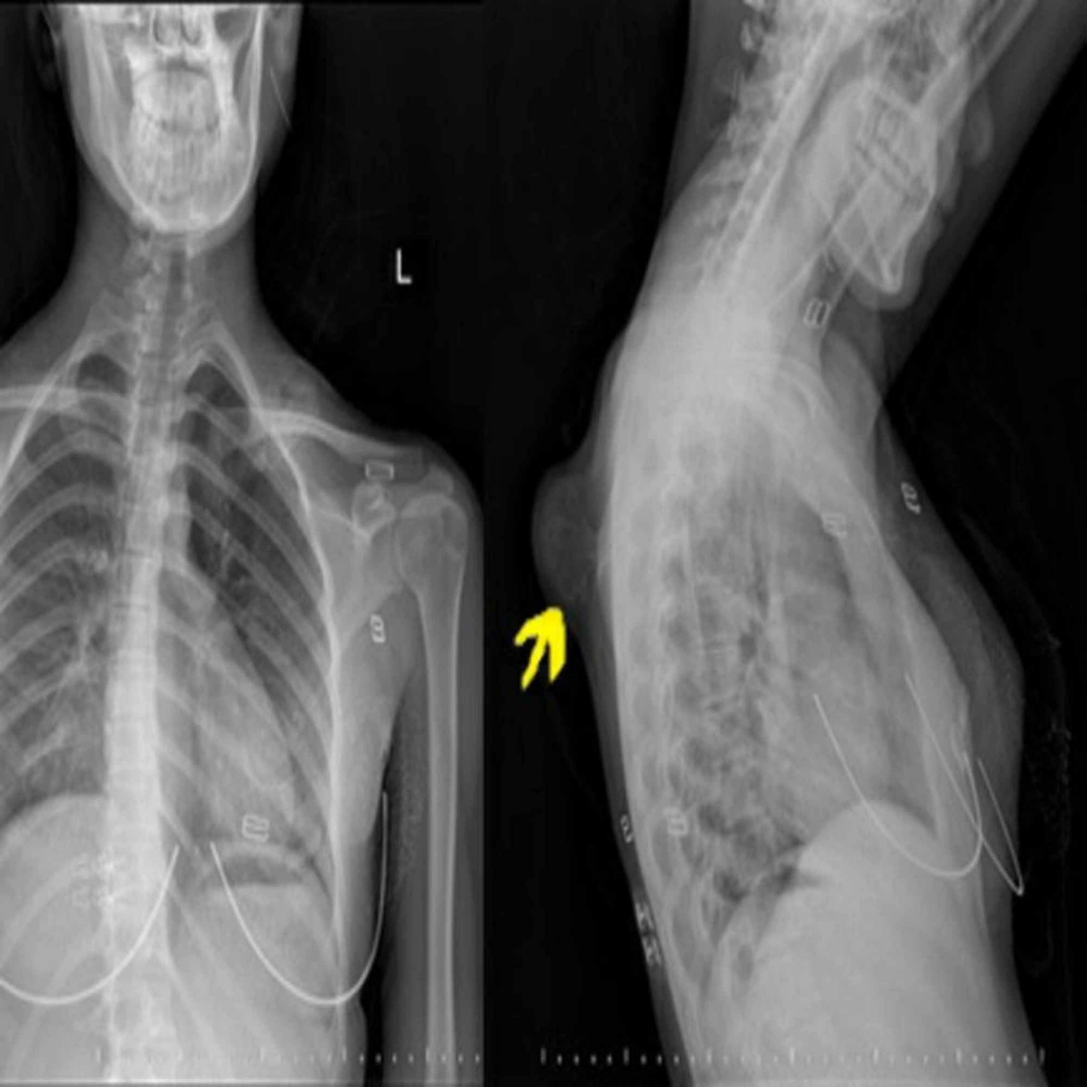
X-rays of the left shoulder revelead a bony mass arising from the dorsal aspect of the left scapula.
